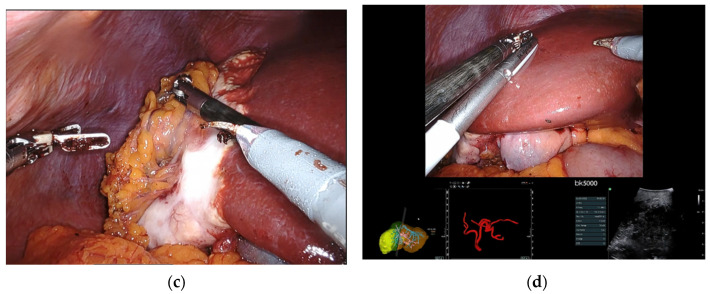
Patient with an AE lesion involving liver segments V/VI/VII/VIII with close contact to the right portal vein and the caval vein, who underwent a right hemihepatectomy including an atypical resection of S IVb. (a) Preoperative CT scan (coronal view); (b) Preoperative PET-MRI (transversal view) demonstrating activity of the lesion in close proximity to the portal vein; (c) Intraoperative image showing the large AE lesion in the right liver lobe; (d) Intraoperative image showing intraoperative resection planning with 3D-reconstructed CT models and intraoperative sonography.
